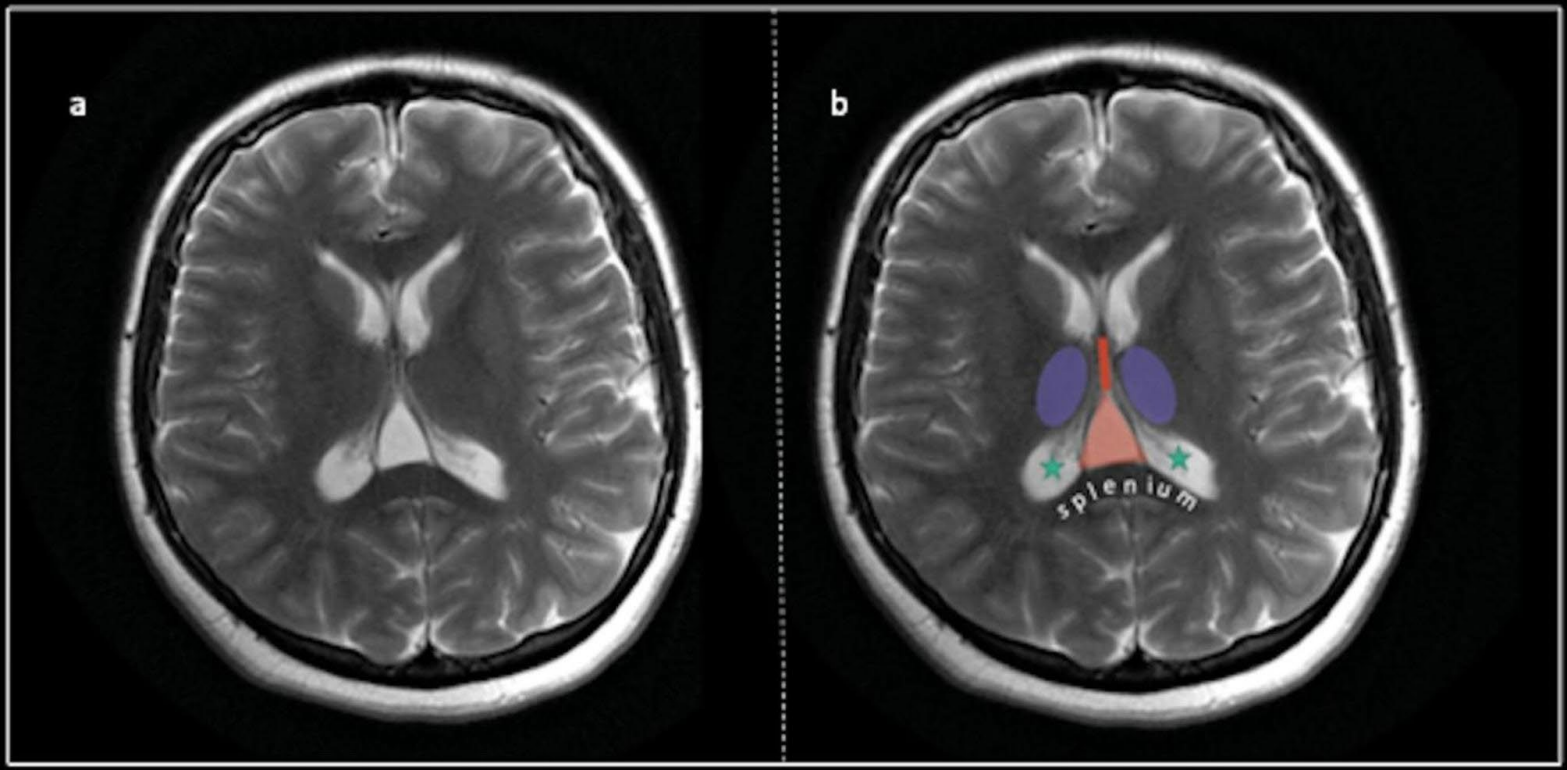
Cavum veli interpositi on brain MRI. *Notes*: Purple: Thalamus; Red: Third ventricle; Triangle-shaped orange area: CVI; Green stars: Lateral ventricles
